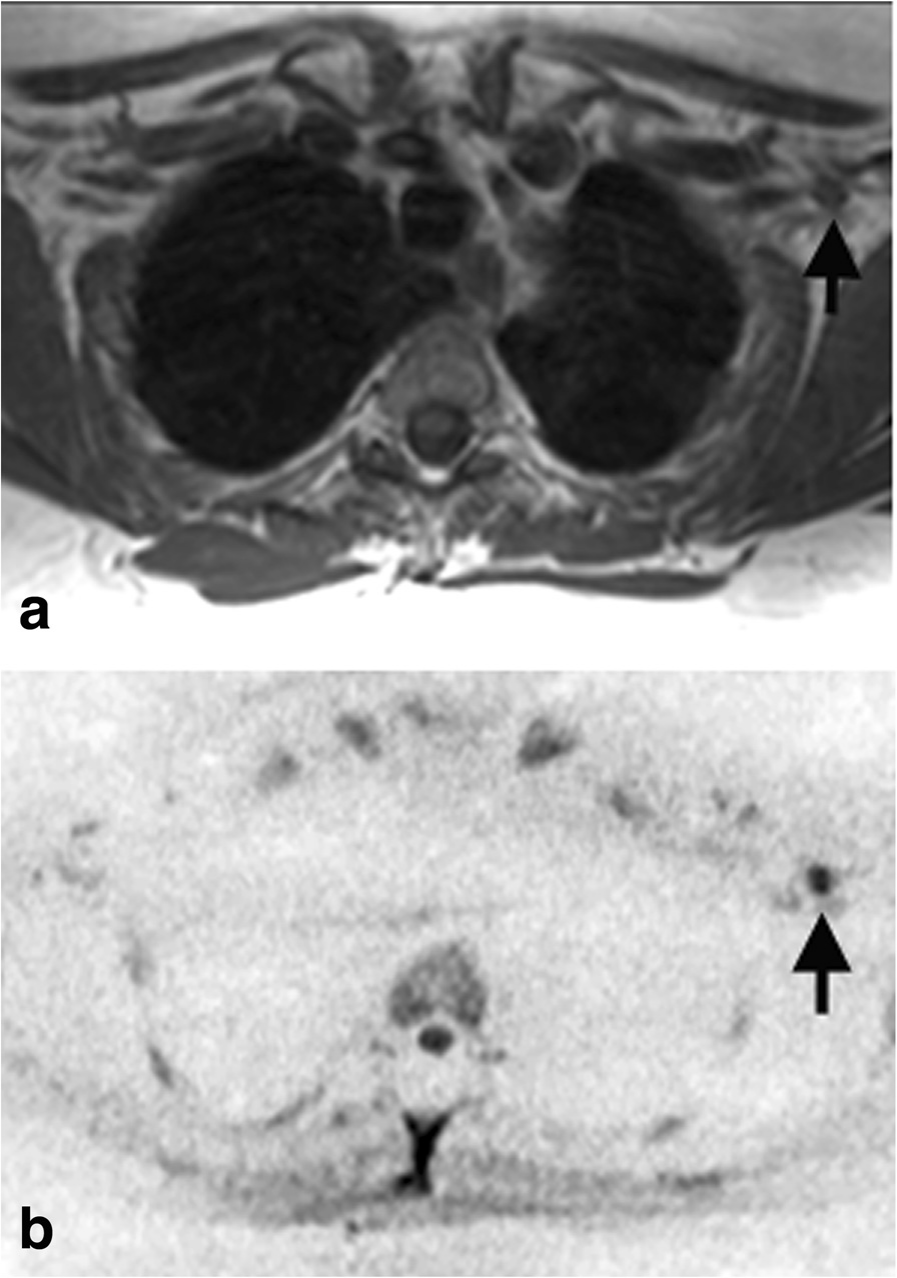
A 52 year-old women with breast cancer. (a) Axial T1-weighted and (b) paraaxial inverted greyscale maximum intensity projection (MIP) of b = 700 s/mm^2^ images. The DW-MRN images show a 4 mm nodular area of impeded diffusion (arrows), which is contiguous with the nerves, in keeping with a neuroma. There was no interval change at follow up imaging. The lesion was overlooked on T1-weighted imaging by one reader.
